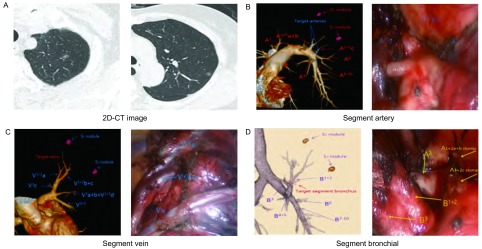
术前三维重建与术中操作。A：2D-CT平扫示左肺尖后段见两结节；B：术前3D-CTBA肺段动脉重建并与术中手术操作对照；C：术前3D-CTBA肺段静脉重建并与术中手术操作对照；D：术前3D-CTBA肺段支气管重建并与术中手术操作对照。术中视频图片由3D转换为2D图片；图中缩写：A为动脉，V为静脉，B为支气管，S为肺段。 Preoperative three-dimensional reconstruction and intraoperative operation. A: 2D-CT scan showed two nodules in the segmentum apicoposterius of the left lung; B: preoperative 3D-CTBA segment artery reconstruction and contrasted with intraoperative operation; C: preoperative 3D-CTBA segment vein reconstruction and contrasted with intraoperative operation; D: preoperative 3D-CTBA segment bronchial reconstruction and contrasted with intraoperative operation. The image of video in the operation is converted to 2D images from 3D; abbreviation in the figure: A: artery; V: vein; B: bronchial; S: segment.
